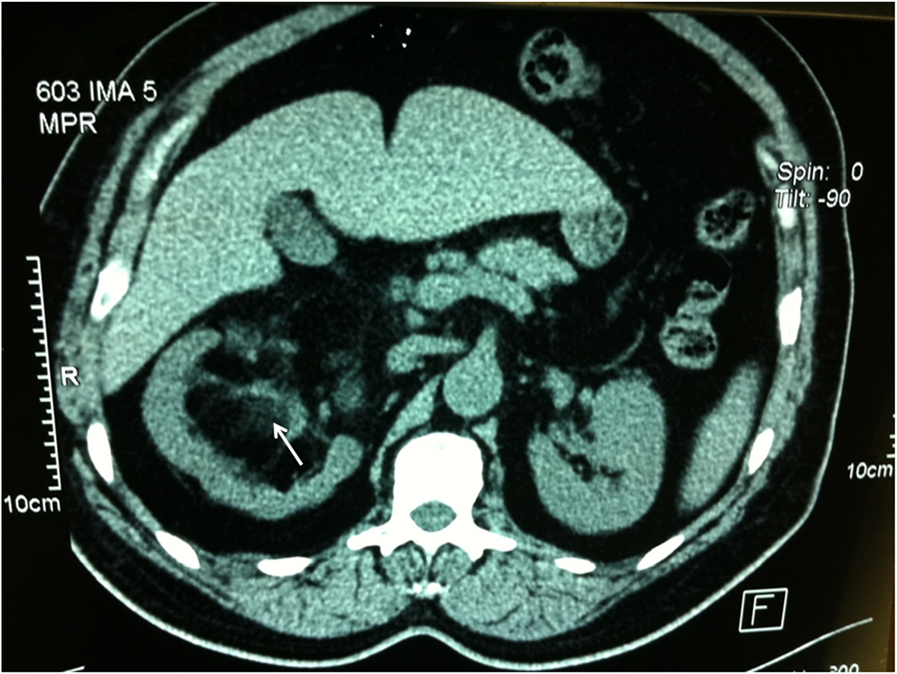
Computed tomography of the abdomen and pelvis showing parenchymal mass of fat density of the right kidney (arrow).
